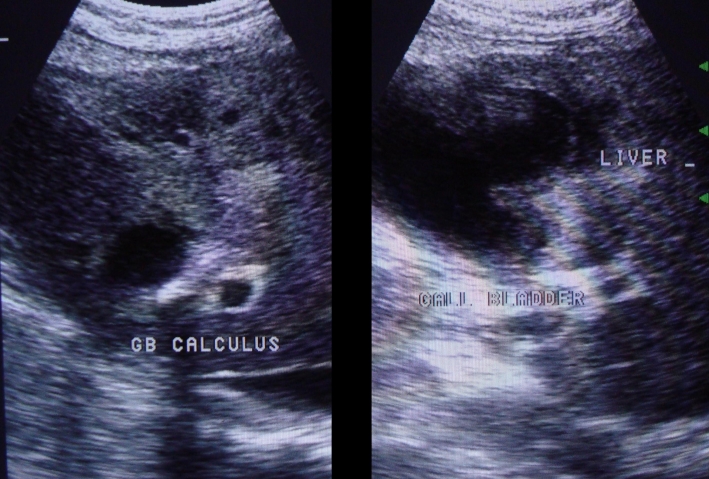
Ultrasonography is showing abscess formation in the liver due to gall bladder perforation
